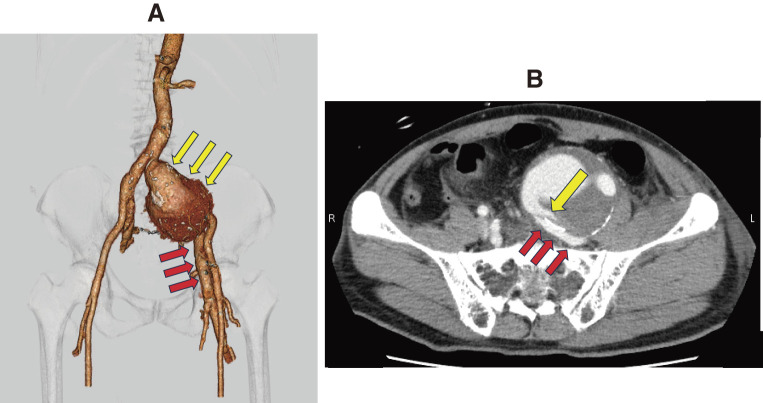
Fig. 1 (A and B) Preoperative computed tomography. Three-dimensional computed tomography reveals a large CIAA (yellow arrows). The distal end of the left CIV (red arrows) markedly enhances in the arterial phase, but the proximal end does not (A). Coronal CT reveals a large shunt between the common iliac artery and vein (yellow arrow). Red arrows demonstrate that the left CIAA compresses the left CIV (B). CIAA: common iliac artery aneurysm; CIV: common iliac vein; CT: contrast-enhanced computed tomography
